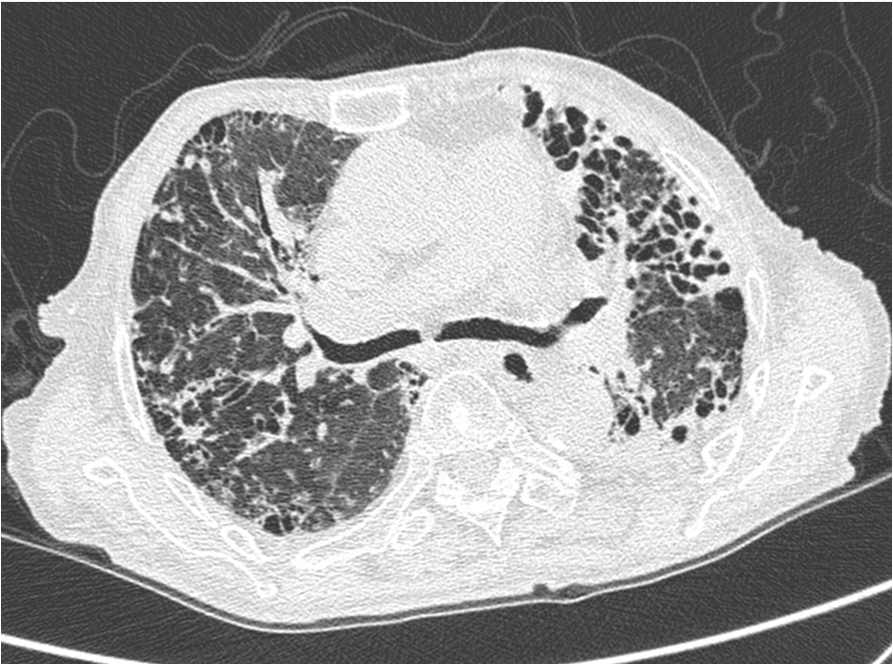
High resolution CT with 1 mm slice-thickness of a 92 –year-old female with NTM (
